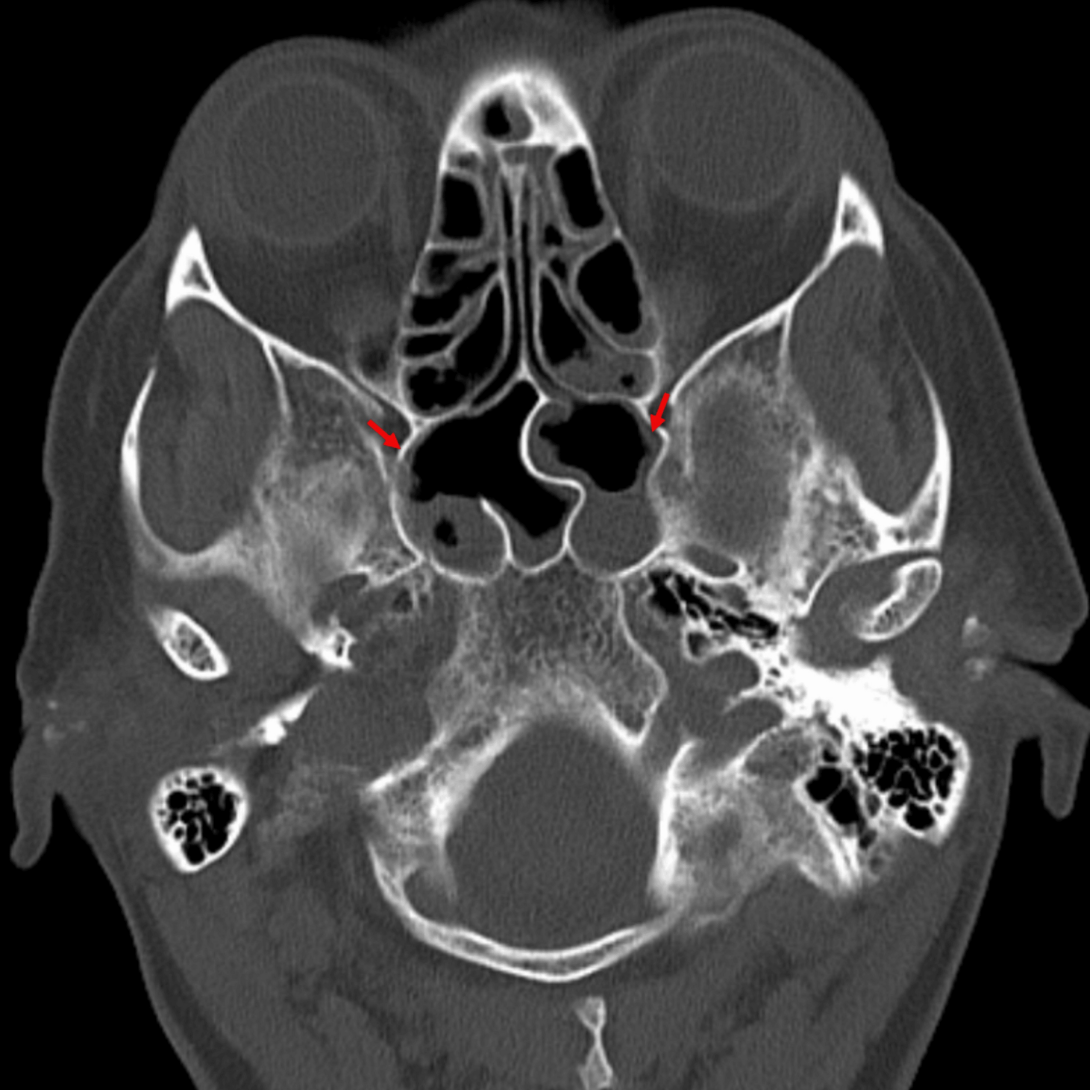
Computed Tomography of the head showing air fluid level in bilateral sphenoid sinus (red arrows)
